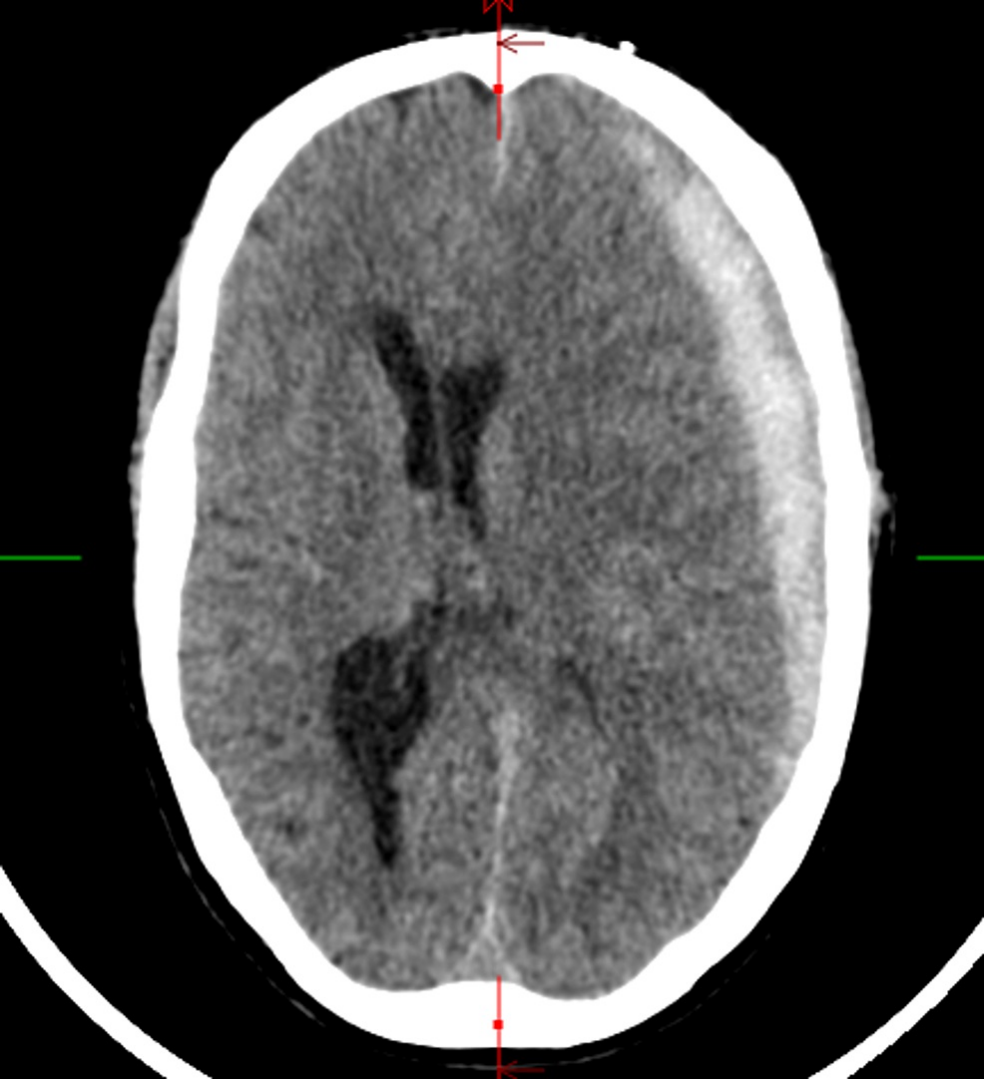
Noncontrast axial head CT at admission showing SDH. SDH = subdural hematoma
